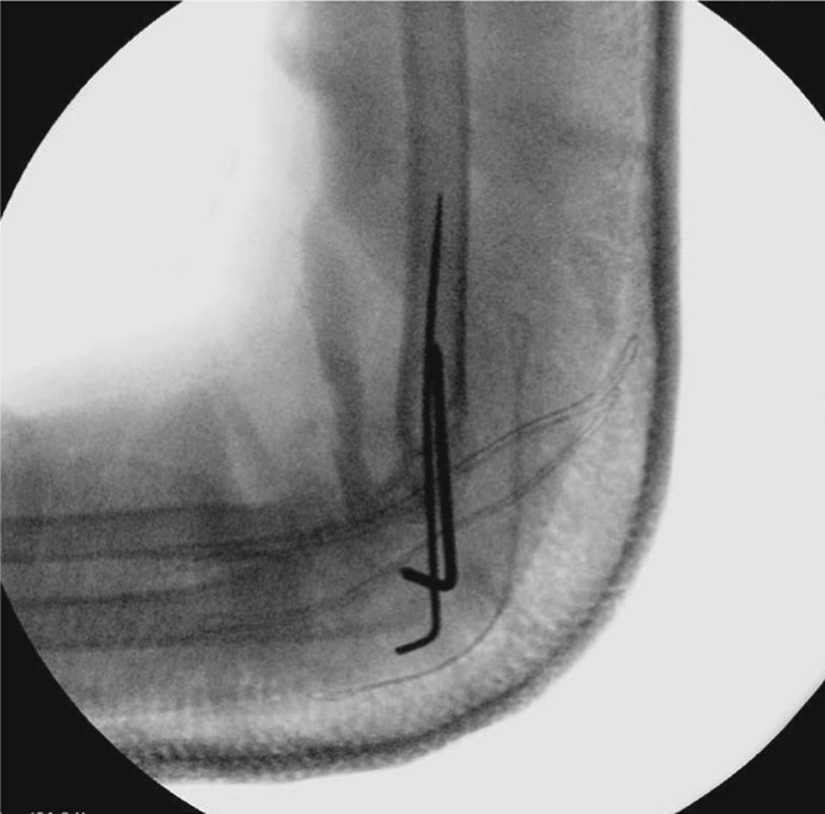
Intraoperative lateral view radiograph: reduction and pinning fixation with two crossed pins
